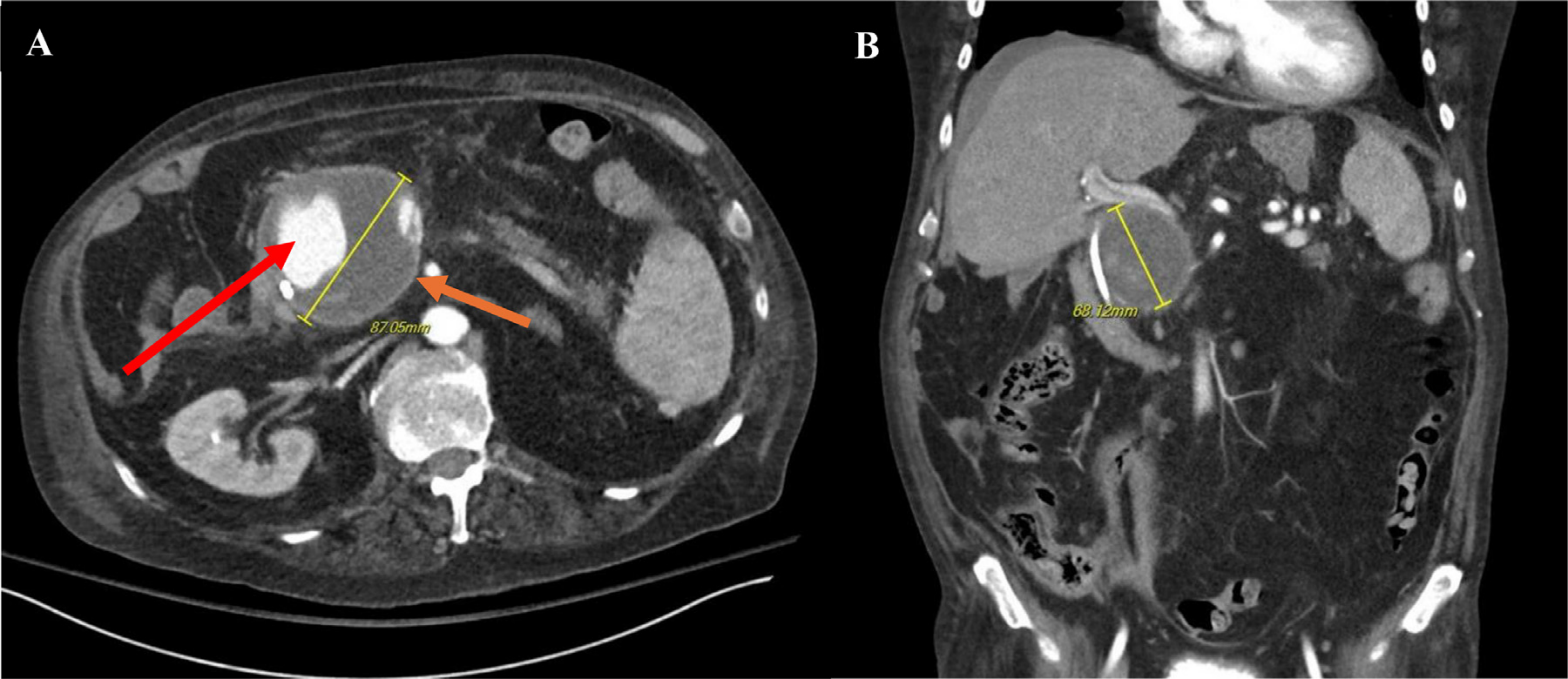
(A and B) The orange arrow highlights a large gastroduodenal pseudoaneurysm, as visualized in both axial and coronal CT images. In the axial plane, the pseudoaneurysm measured 87.05 mm, while in the coronal plane, it measured 68.12 mm. The red arrow indicates the presence of radiopaque contrast material within the pseudoaneurysm cavity and the surrounding hypodense region represents chronic thrombosis.
